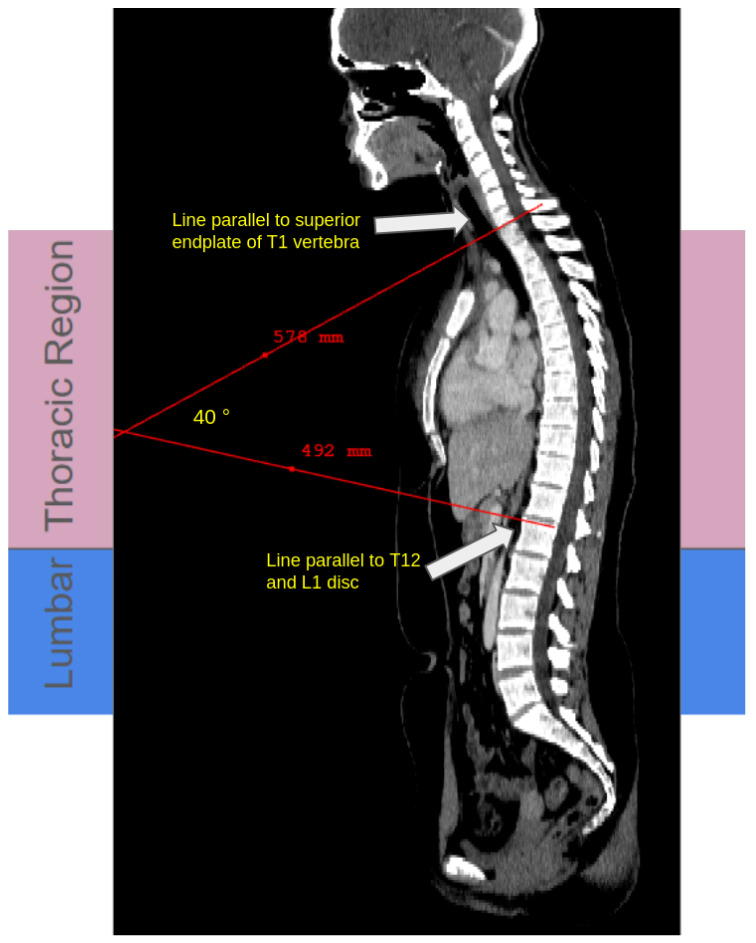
Manual thoracic supine kyphosis measurement on CT. Representative mid-sagittal CT reconstruction showing the thoracic and lumbar regions, with red lines drawn parallel to the superior endplate of T1 and the inferior endplate of T12/L1. The intersection of these red lines defines the supine Cobb angle, representing the degree of residual thoracic supine kyphosis with supine positioning [1]. Regional color overlays indicate the thoracic (pink) and lumbar (blue) segments. Measurements and visualization were performed using ITK-SNAP [23]. The same convention was applied across the full internal cohort (n=533) used for inter-observer reliability analysis.
